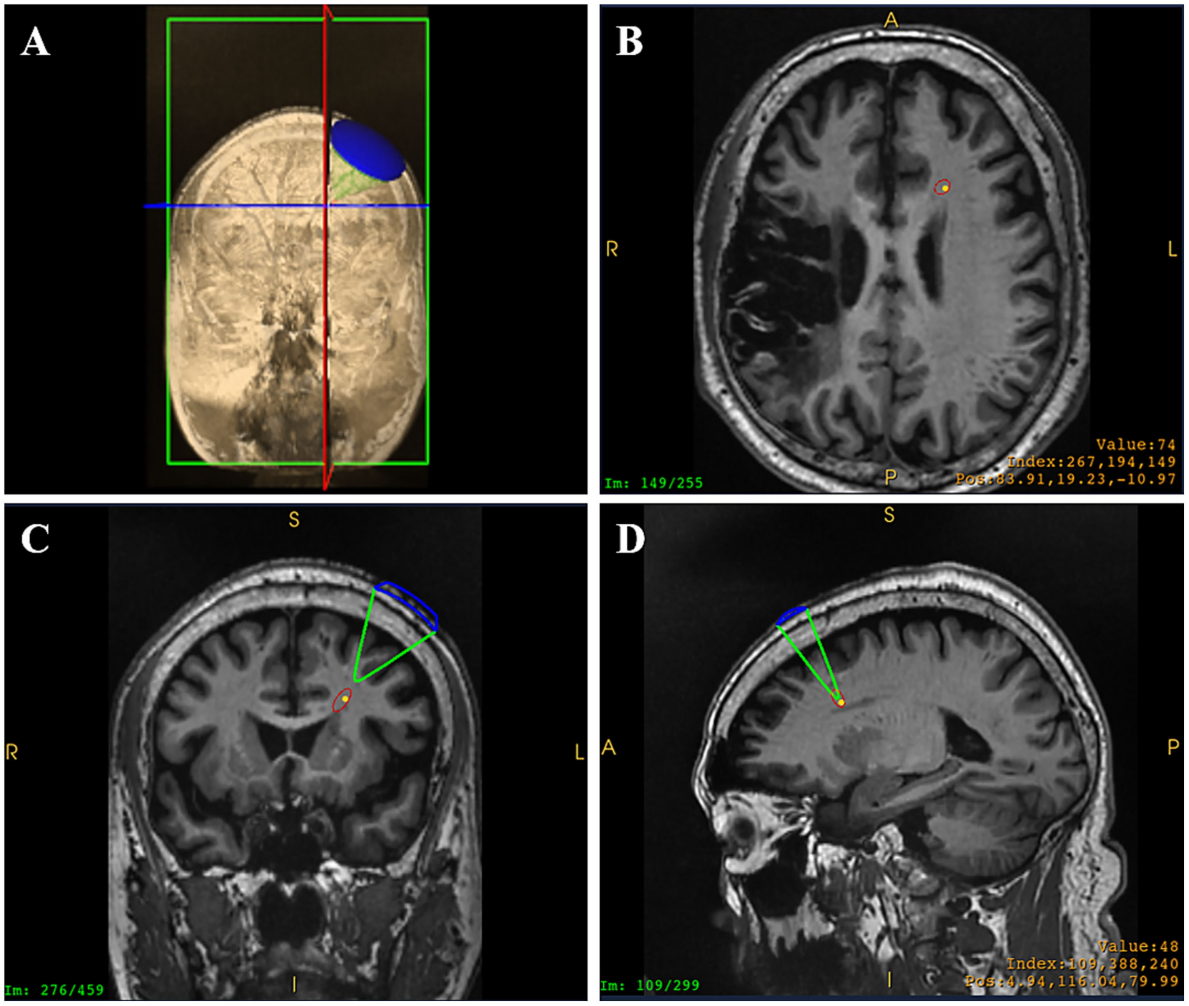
Transducer placement (A) and real-time monitoring of tLIFU focus in the horizontal plane (B), the coronal plane (C), and the sagittal plane (D) within the therapeutic session (the yellow dot represents the preset neuromodulation target, and the red ellipse represents the focus of the ultrasound transducer. A, anterior; F, feet; H, head; I, inferior; L, left; P, posterior; R, right).
